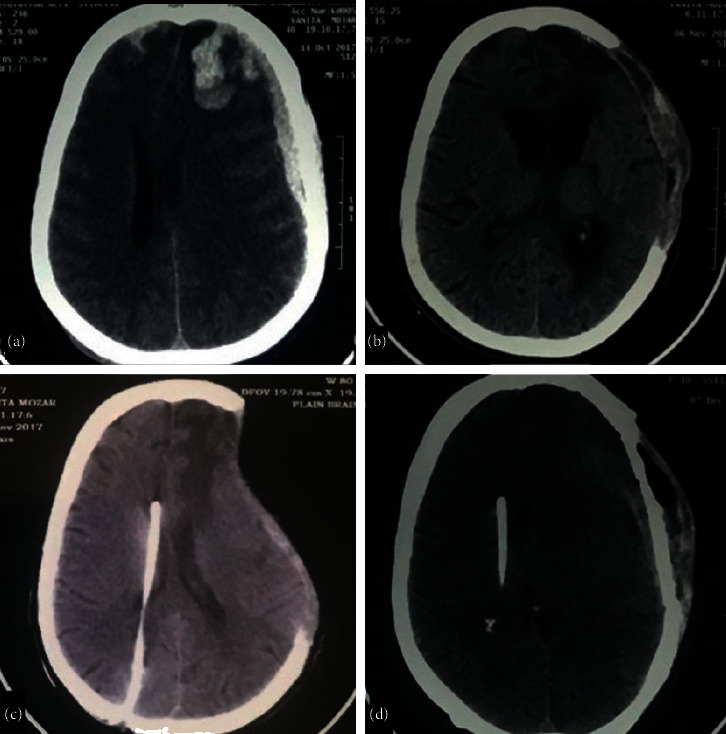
(a) Computed tomography (CT) plain brain, axial view showing hyperdense collection along left subdural plane extending across frontal, temporal, and parietal regions. (b) Computed tomography (CT) plain brain, axial view showing dilated ventricles and herniation of brain from craniectomy site. (c) Computed tomography (CT) plain brain, axial view showing VP shunt in situ and excessively sunken brain at craniectomy site causing midline shift to right side. (d) Computed tomography (CT) plain brain, axial view on postoperative day 1, showing cranioplasty using flattened bone. Also, partial resolution of midline shift can be seen.
